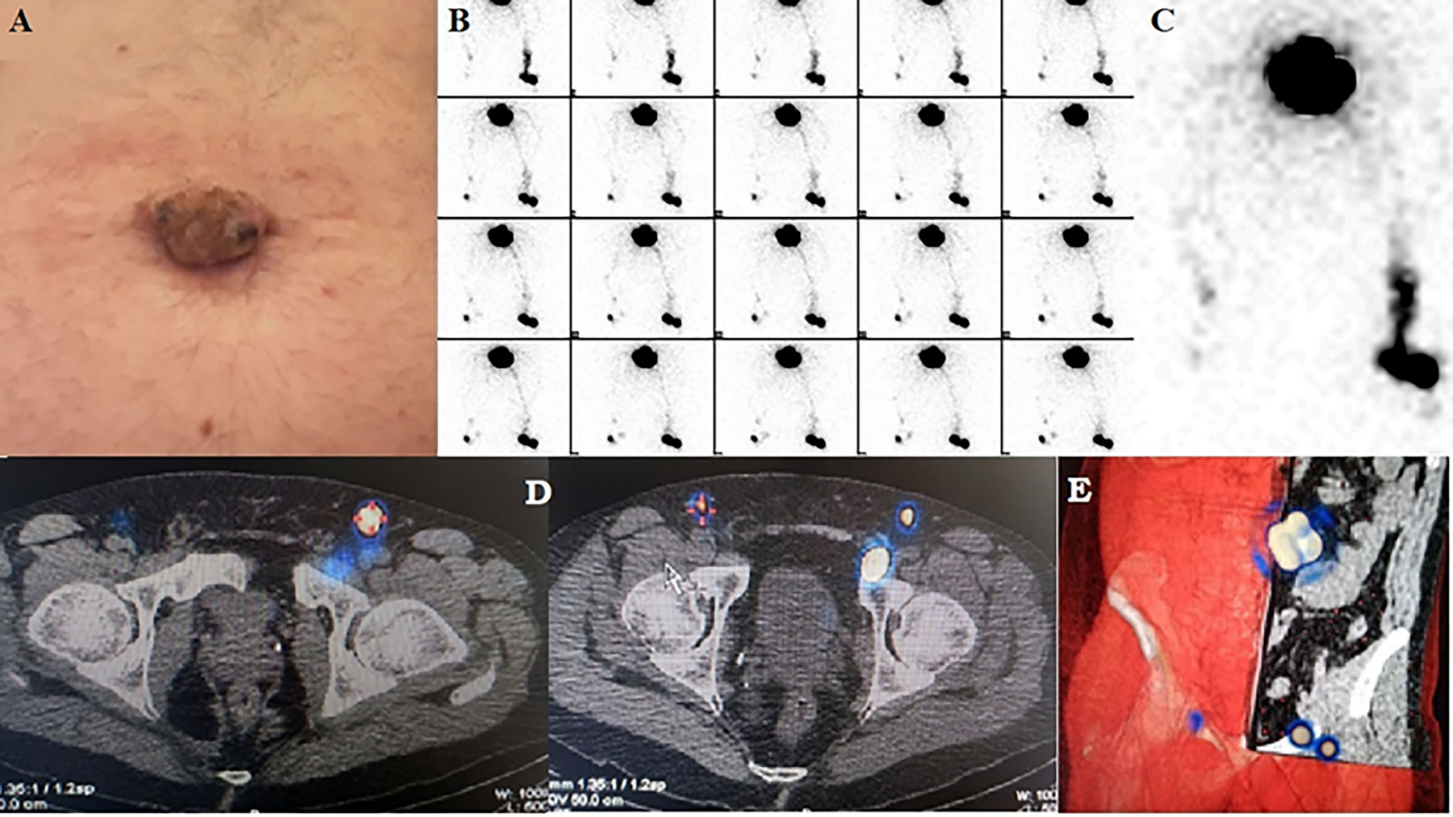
(A) Umbilical melanoma (B) Dynamic sequential images showing three lymphatic collectors departing from peritumoral injection sites (C) Detail showing direct drainage from the injection site to the left external iliac lymph node. (D) Fused axial SPECT-CT sections differentiate inguinal sentinel nodes from an external iliac sentinel node on the left. (E) Volume rendering SPECT-CT of the true sentinel nodes used for guiding surgery.
